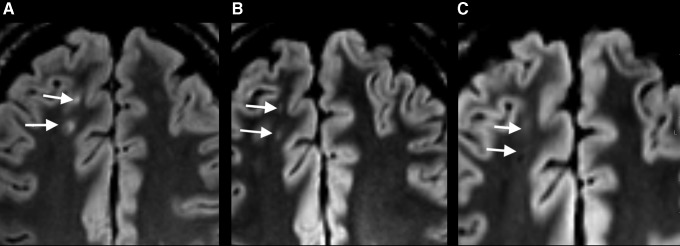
(A–C) Traumatic axonal injury (TAI) on fluid attenuated inversion recovery (FLAIR) scan in the transverse plane of the same patient as in Figure 3, acquired at three different time points. (A) Hyperintense lesions (arrows) at the gray-white matter junction in the right frontal lobe on the very early FLAIR scan. (B) At 3 months, the lesions were less conspicuous. (C) At 12 months, the lesions appeared even more isointense with surrounding normal white matter tissue.
